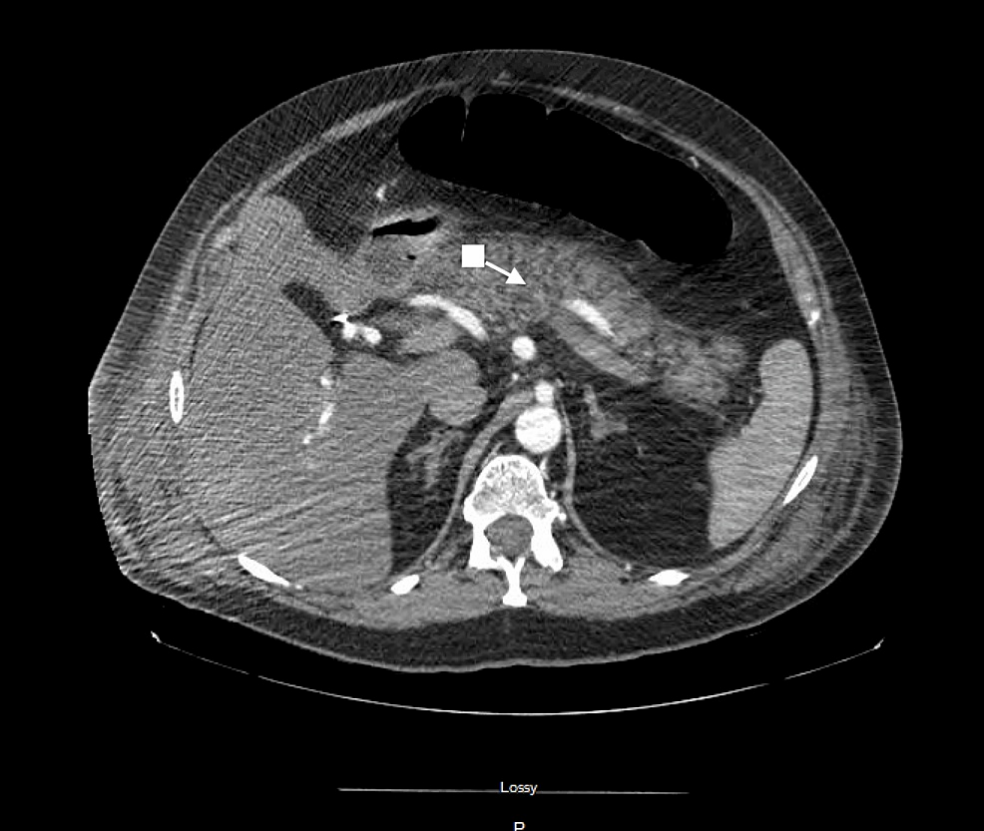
CT showing inflamed pancreas (arrow) CT: computed tomography
